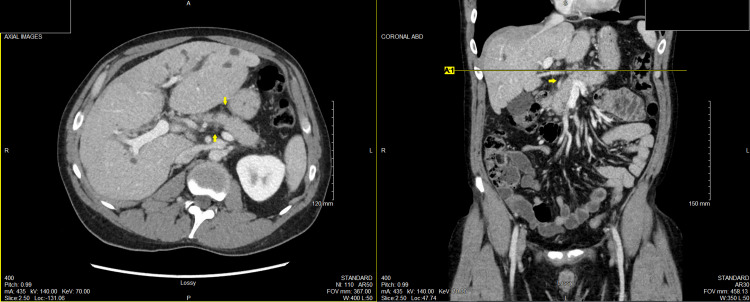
CT A/P with acute interstitial pancreatitis, fat stranding, and edema around the pancreatic parenchyma.
